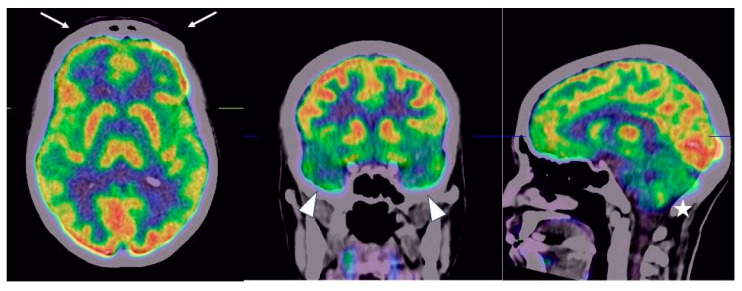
Fused 18F-FDG PET/CT images on axial, coronal and sagittal planes confirmed the reduction of tracer distribution in the aforementioned cerebral regions, inferior frontal lobe (olfactory gyrus) (arrow), in the temporal lobe (limbic/paralimbic regions) (arrowhead) and in the cerebellum (star). On the co-registered CT image, no-specific alterations of cerebral parenchyma were observed.
